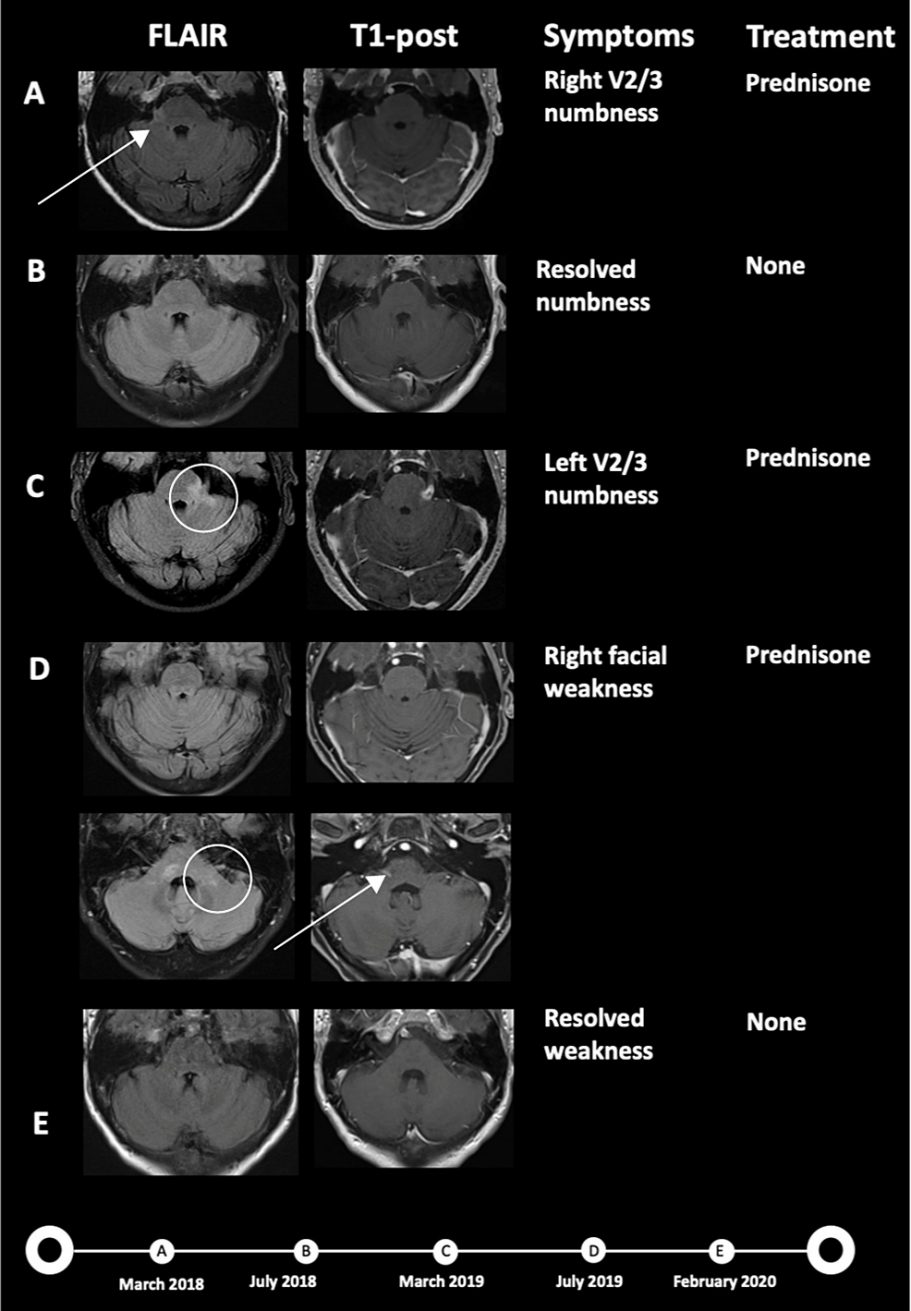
Clinical presentation: Imaging, symptoms, treatment, and timeline. The patient initially presented with right V2/3 sensory changes and was found to have fluid-attenuated inversion recovery (FLAIR) signal abnormality and enhancement at the trigeminal root entry zone (Arrow A). Symptoms improved after prednisone monotherapy and follow-up imaging showed resolution of FLAIR signal abnormality and enhancement (B). She later experienced new left-sided V2/3 sensory changes and was found to have an abnormal FLAIR signal and enhancement of the left trigeminal root entry zone (Circle C), treated with prednisone (C). She later developed a right facial palsy, treated with steroids. MRI revealed FLAIR signal abnormality and abnormal enhancement (Arrow D) involving the cisternal, canalicular, and labyrinthine segments of the right facial nerve. Previous FLAIR signal abnormality in the left trigeminal root entry zone (Circle D) resolved (D). Her facial weakness and FLAIR signal abnormality resolved on follow-up imaging (E). There was no abnormal signal in the right parietal lobe from March 2018 to February 2020 (not shown).
